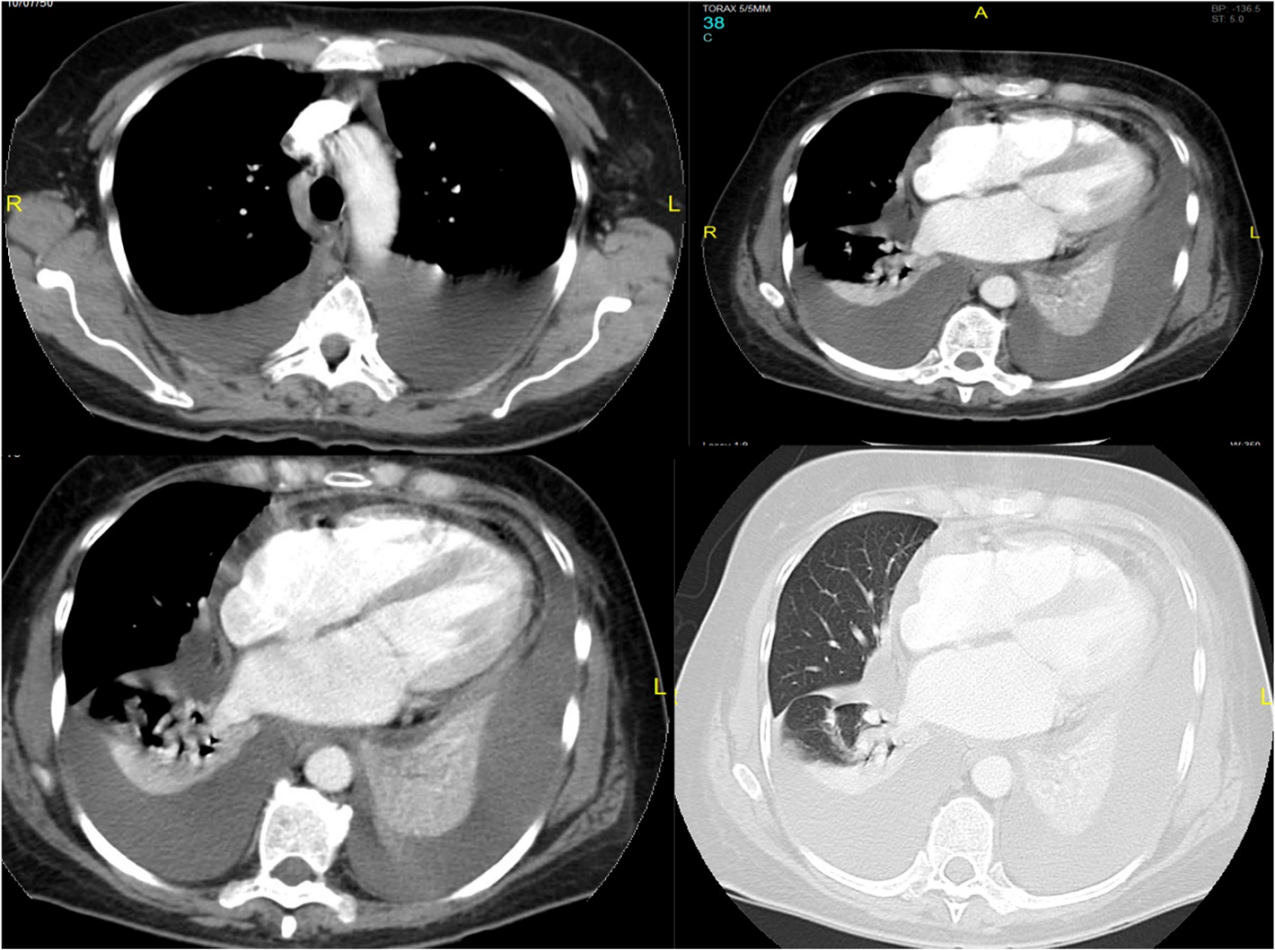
Different aspects of the thoracic computed tomographic scan showing bilateral pleural effusion, thickening of the pericardium, and pericardial effusion, without evidence of enlarged lymph nodes or pulmonary infiltrates. *R* right side, *L* left side
